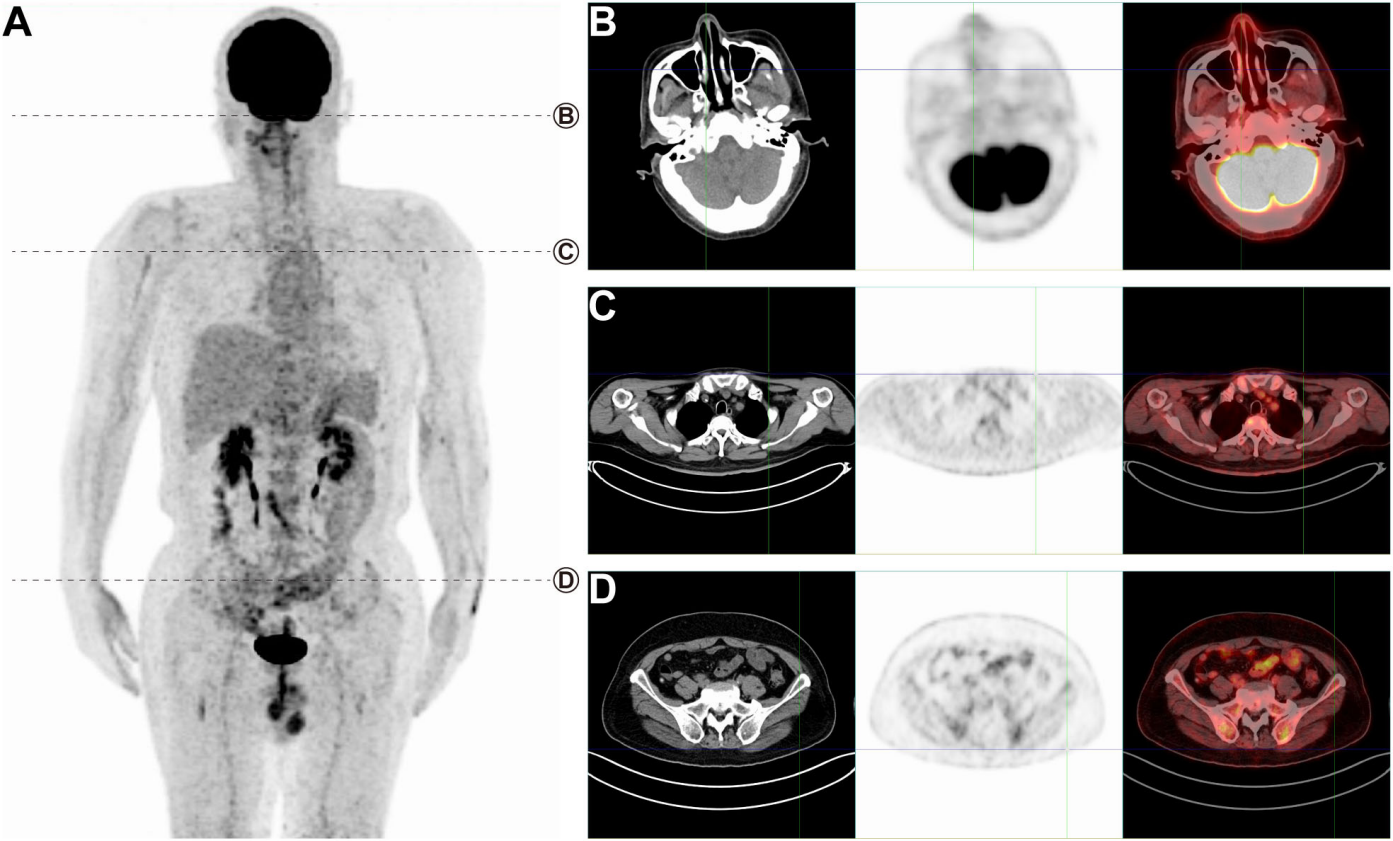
PET/CT imaging after treatment. (A) A maximum intensity projection (MIP) whole-body image. (B–D) No abnormal uptake from the nasal cavity or skin.
